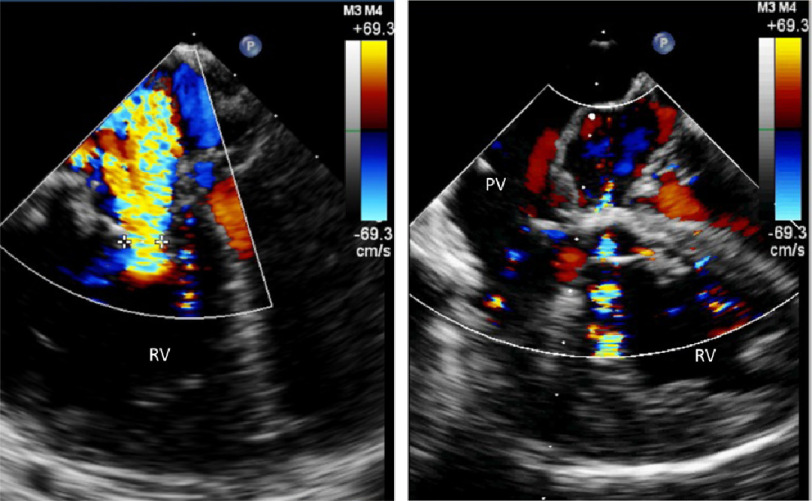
A. Pre-operative color doppler showing severe TR. B. Post-banding image with trivial TR (arrow). RV: Right ventricle, PV: Pulmonary venous channel.
